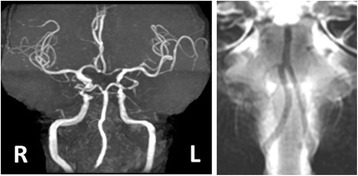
Magnetic resonance angiography revealing the disappearance of the left vertebral artery (left panel). Basi-parallel anatomic scanning magnetic resonance imaging delineates the outside shape of the left vertebral artery, indicating that the vertebral artery was not hypoplastic but obstructed (right panel)
